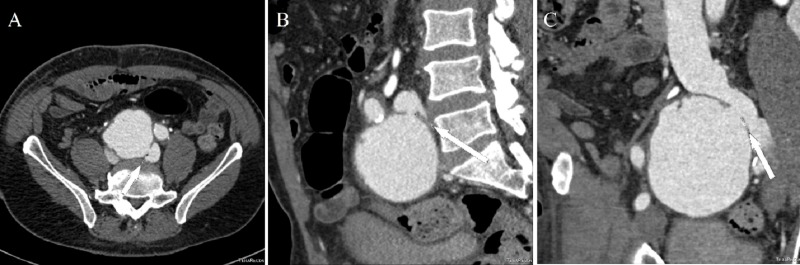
A, B, C Axial, coronal, and sagittal oblique computed tomography angiography (CTA) images through the pelvis demonstrate a fistulous tract (arrows) between an aneurysmal dilatation of the right common iliac artery and the adjacent left common iliac vein with early enhancement of the latter.
